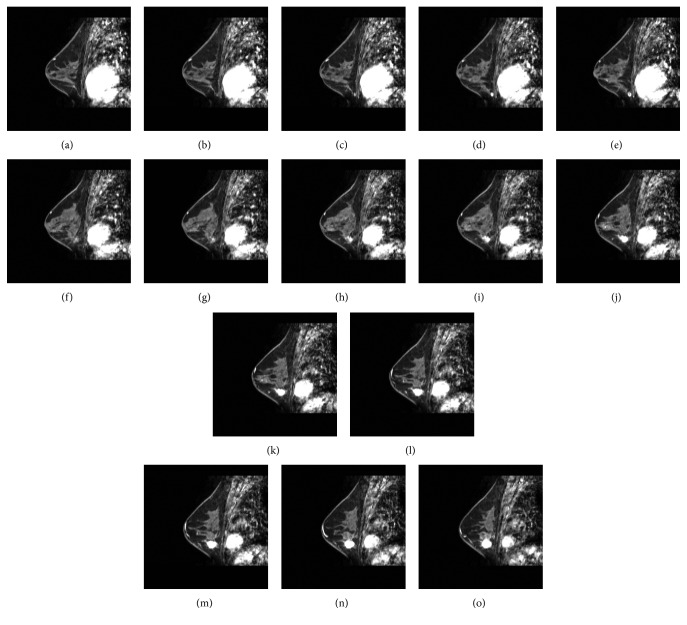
A partial image slice of an MRI case with a breast tumor.
